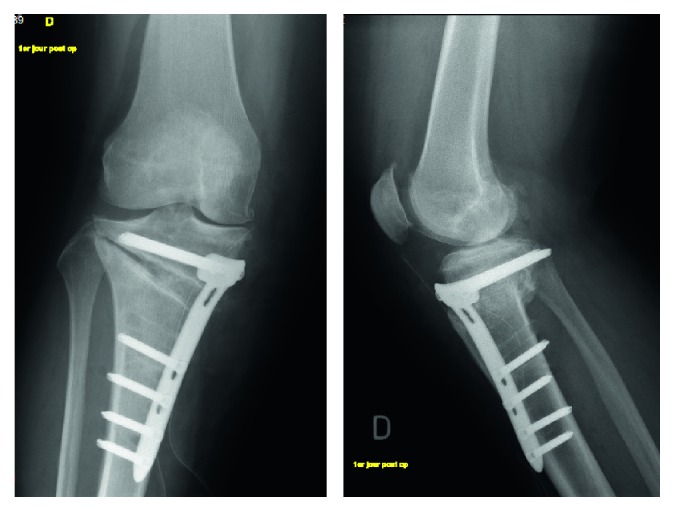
June 2010, postoperative X-rays after revision.
